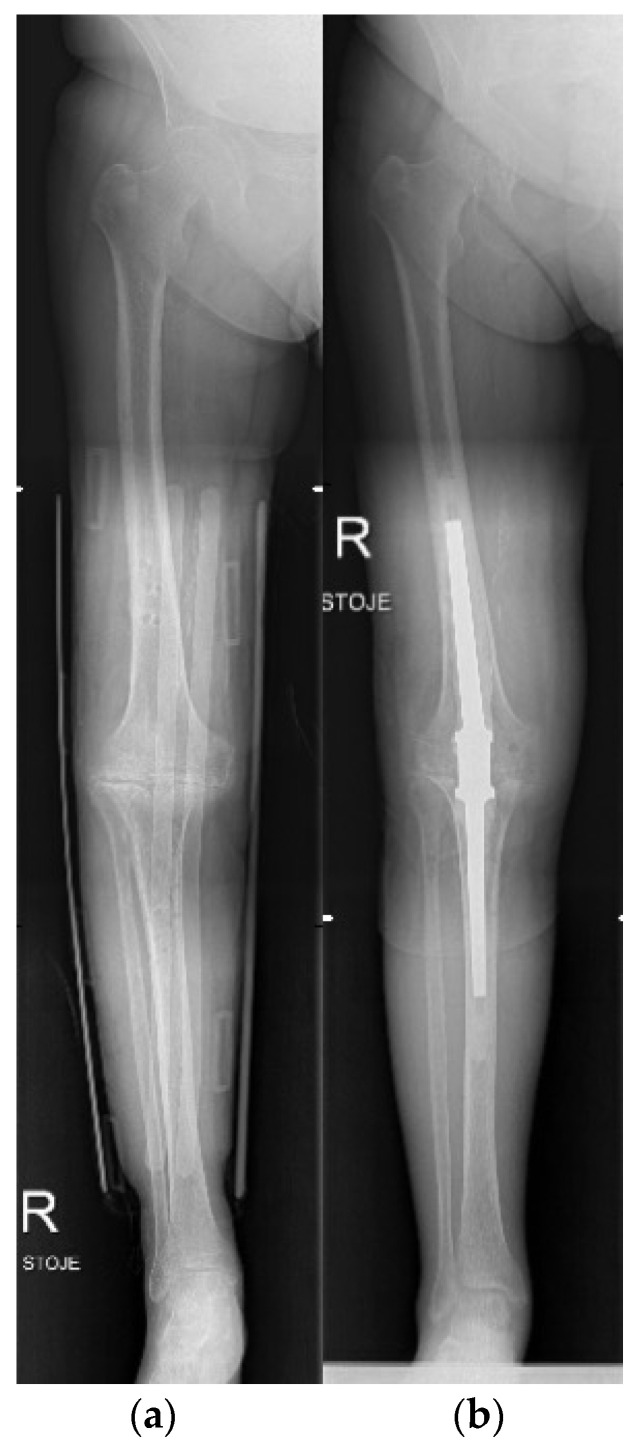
Knee arthrodesis. (a) preoperative X-ray in a splint; (b) postoperative X-ray after limb salvage knee arthrodesis with a nail.
